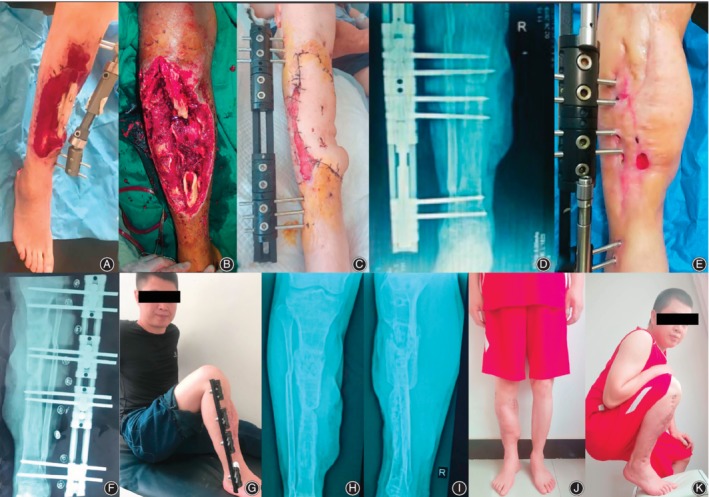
An 38‐year‐old male patient with posttraumatic tibial defect associated with soft tissue defect treated with radial debridemnt, followed by free latissimus dorsi flap transfer and trifocal bone transport using LRS from proximal to distal. (A) Soft tissue defect with tibial shaft exposure after index surgery. (B, C) Removal of devitalized bone and soft tissue by excisional debridement and latissimus dorsi flap is transferred to the coverage of soft tissue defect with OLRS placed on anteriolateral side. (D, E) Trifocal bone transport was completed with successful flap survival in converging direction. (F) Consolidation of regenerates after docking in 3 months. (G, H, I) Functional and radiographic result showed complete consolidation of regenerates on AP view before removal of external fixator. (G, K) Functional recovery of patients showed acceptable range of motion of knee and ankle joint at last visit.
